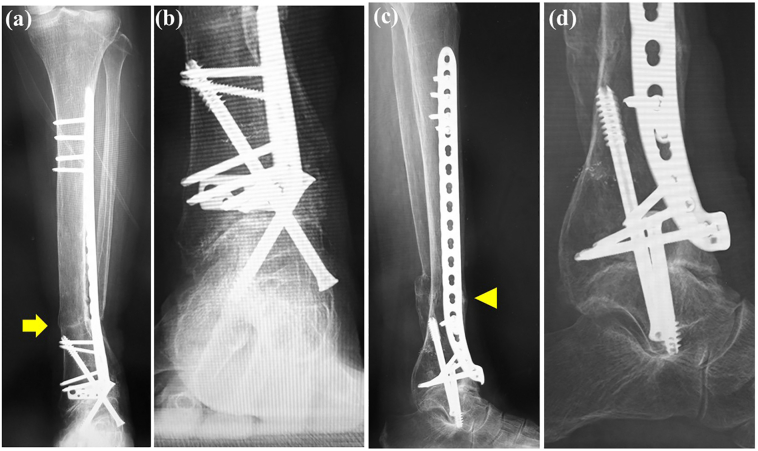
Standing anteroposterior radiographs of the (a) lower leg and (b) ankle obtained at the 2-year follow-up visit show correction of the valgus deformity of the tibia with complete bony consolidation at the sites of the corrective osteotomy and ankle fusion without dislocation or failure of the implant (arrow). Standing lateral radiographs of the (c) lower leg and (d) ankle obtained at the 2-year follow-up show correction of the recurvatum deformity of the tibia (arrowhead).
